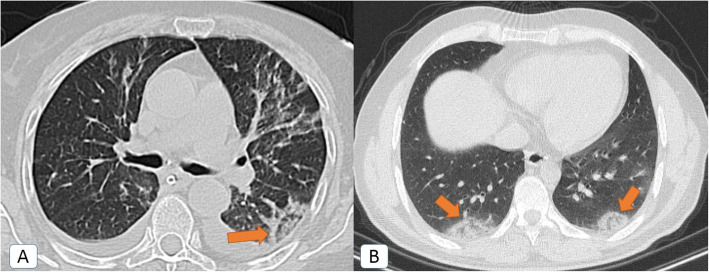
Bulls-eye sign or target sign or double-halo sign: a 36-year-old male COVID-19 patient. A, B Axial chest CT (lung window) showing bilateral posterior basal sub-pleural patches of central and peripheral consolidations with intervening ground-glass attenuation. This is together with bilateral minimal pleural collection and left lingular mild fibro-atelectatic bands
